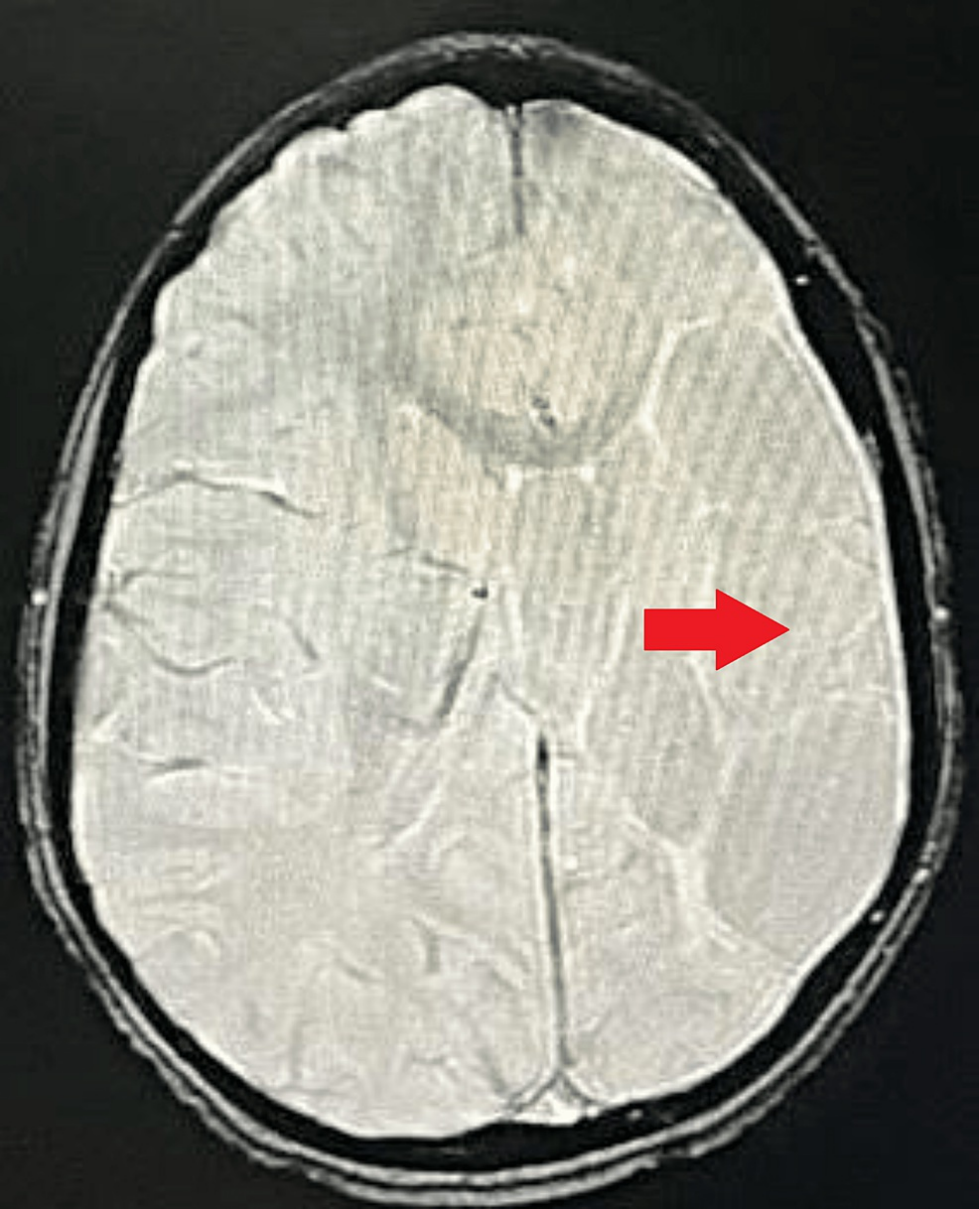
MRI brain axial SWAN image No evidence of blooming is noted Red arrow: no evidence of blooming at the left frontoparietal lobe SWAN: susceptibility-weighted angiography
